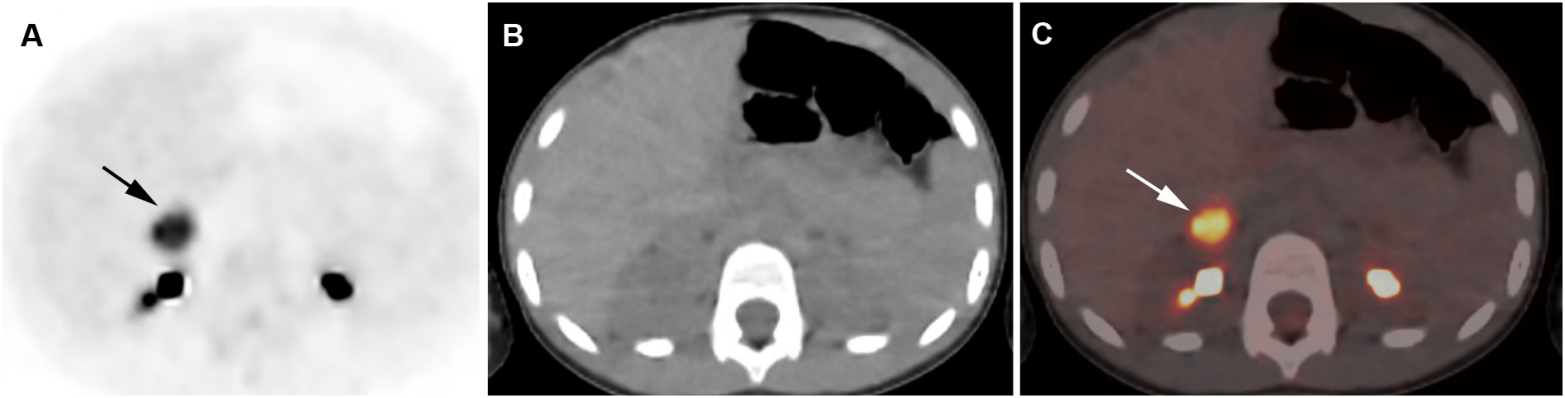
Adrenocortical adenoma. 5-year-old girl with history of neuroblastoma 4 years earlier. Suspected recurrence of neuroblastoma. Adrenal lesion noted on ultrasound as part of an evaluation of the urinary tract. [^18^F]FDG PET shows uptake in a right adrenal mass. Surgical resection of 2.5-cm adrenal adenoma. Transverse PET (A), CT (B), PET/CT fusion image (C). Intense [^18^F]FDG uptake in a right adrenal mass (arrows).
